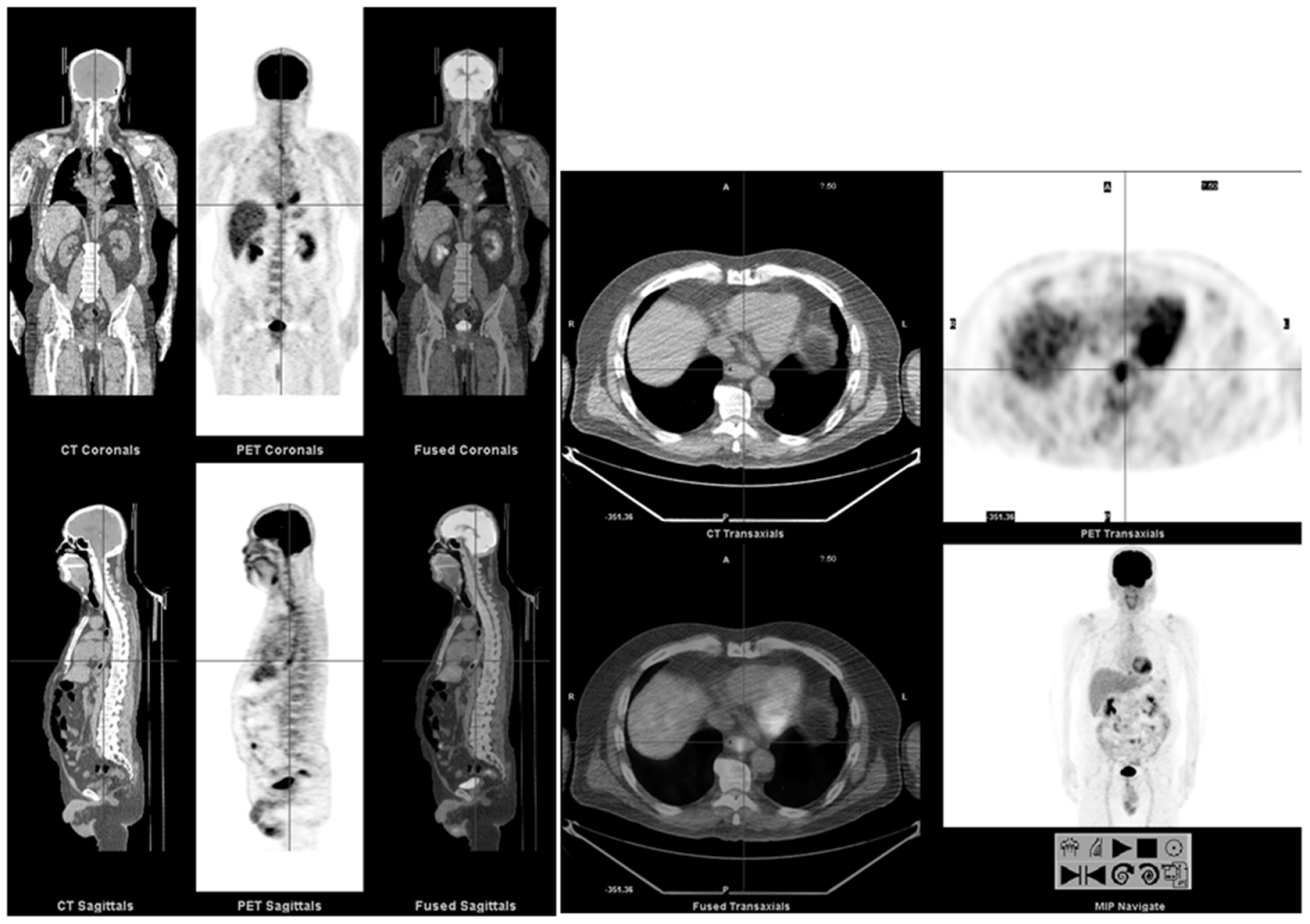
FDG PET/CT images from a 59-year-old male with Barrett's esophagus. PET/CT showed intense FDG accumulation with correlative wall thickening in the esophagogastric junction (cursor, SUV_max_ = 5.6).
